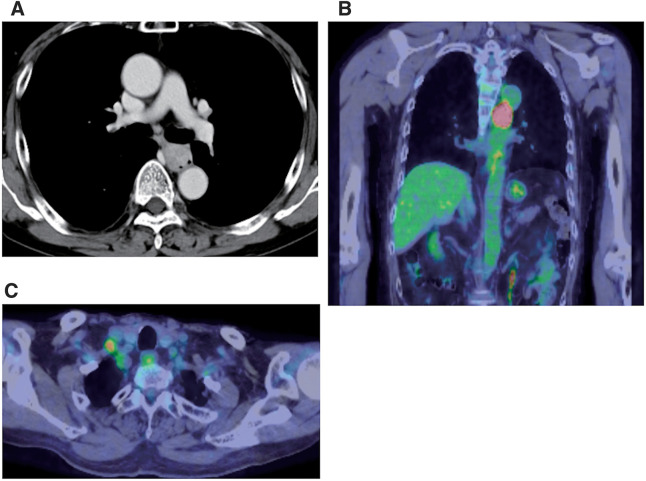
Preoperative findings. (A) Contrast-enhanced computed tomography of chest showing a mass lesion with contrast effect in the middle thoracic esophagus. (B) Fluorodeoxyglucose positron emission tomography-computed tomography showing an abnormal accumulation in the same region. (C) Accumulated lymph node (Station No. 104R).
